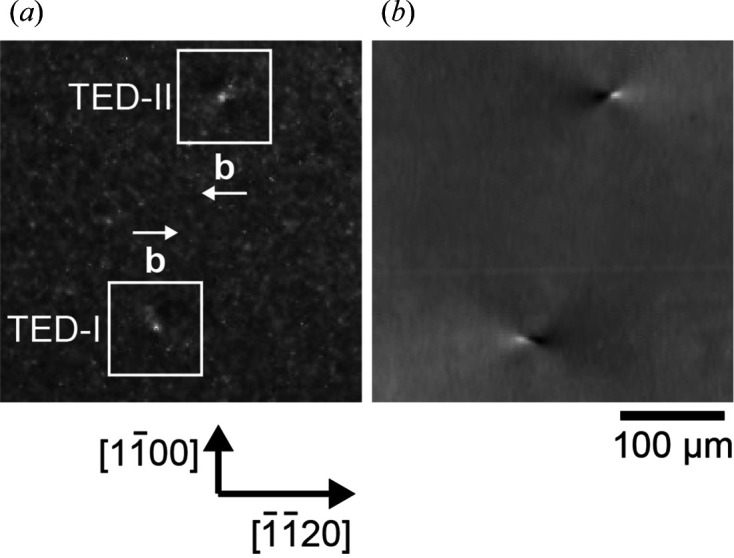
(*a*) X-ray topography image and (*b*) birefringence image under conditions slightly deviating from crossed Nicols (ɛ = 5°) taken at the same position on an SiC wafer (Δ*l* = 150 µm). The direction of the Burgers vector for TED-I is [
